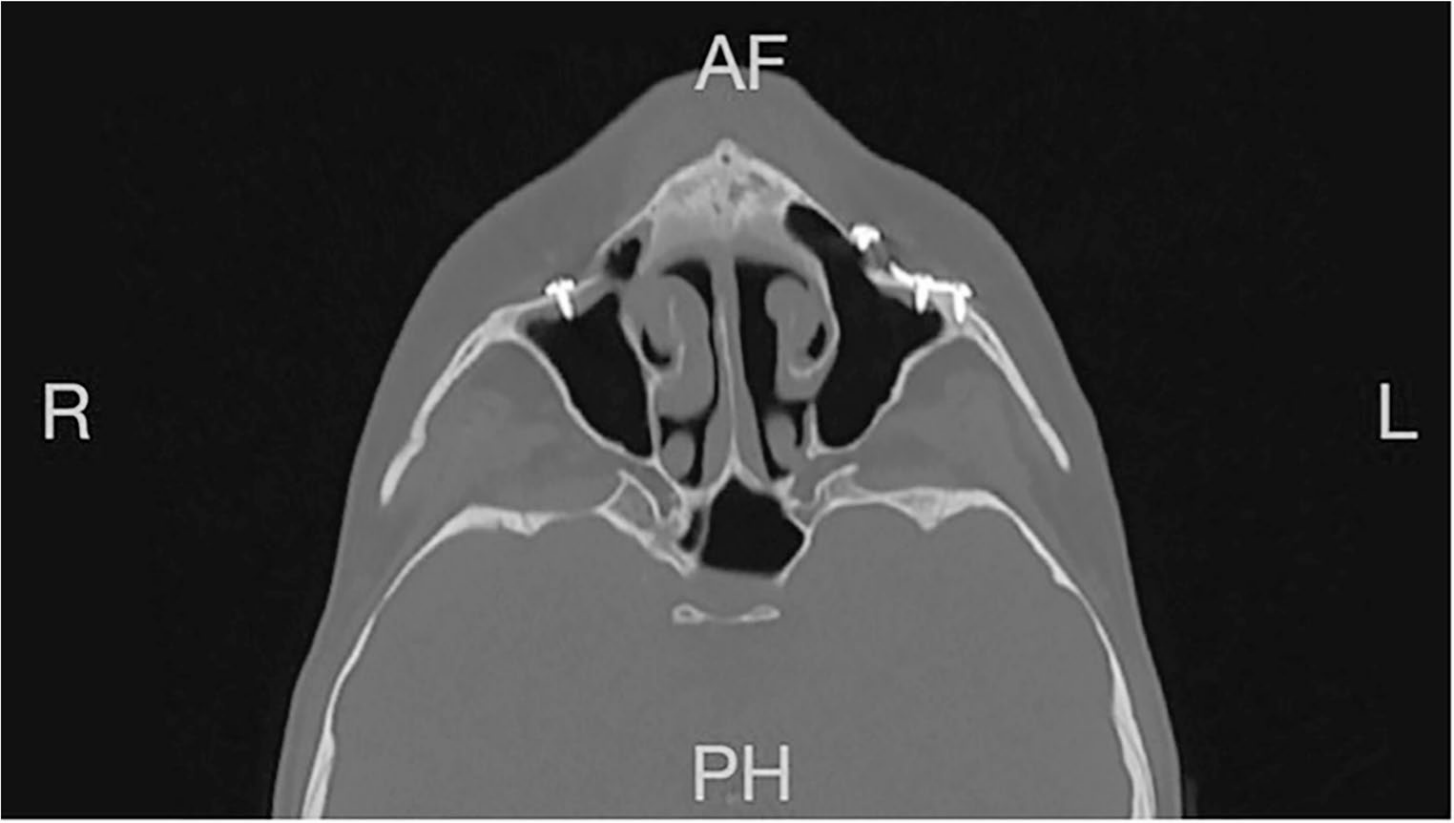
Postoperative axial CT scan showing mucosal thickening in a patient with excessive screw length
